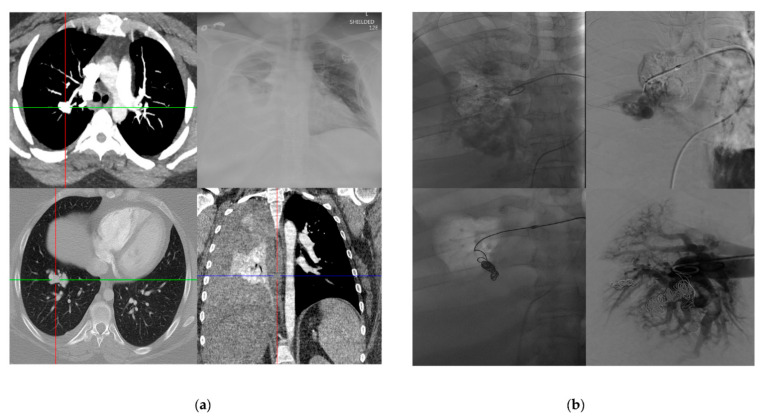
A 40-year-old female with HHT who presented with hemoptysis. (a) CXR showing large left-sided hemothorax, confirmed on CT, causing collapse of the adjacent lung. (b) Pulmonary angiography demonstrating a complex RLL AVM, followed by coil embolization and resolution of bleeding.
